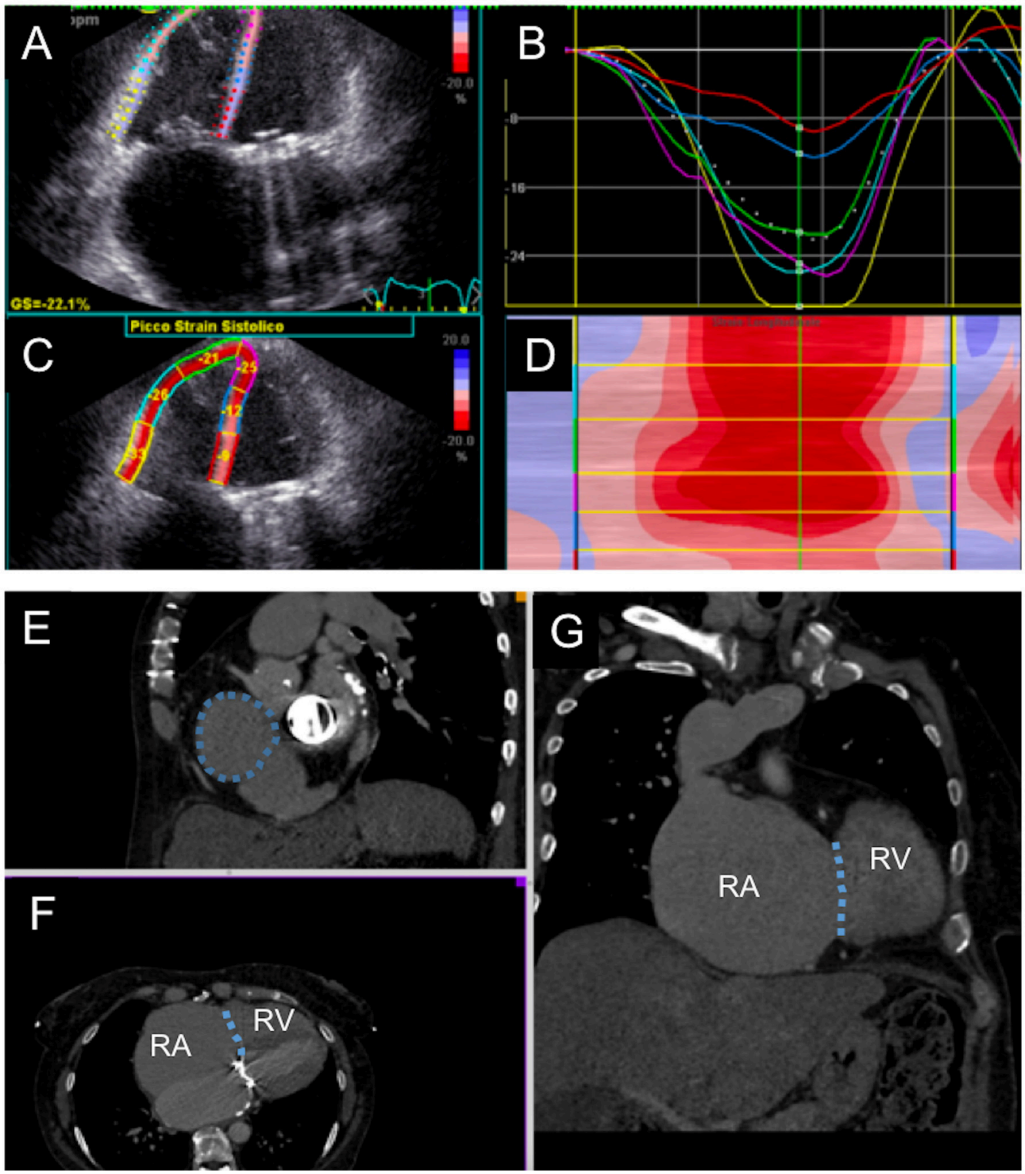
Multimodality evaluation for percutaneous tricuspid valve replacement. (A–D) Echocardiographic evaluation of right ventricular function with semi-automated strain measurement. (E–G) Computed tomography cardiac chamber and tricuspid valve evaluation; the patient presented with severe right ventricular (RV) and right atrial (RA) dilatation; tricuspid annulus is highlighted with dotted line.
